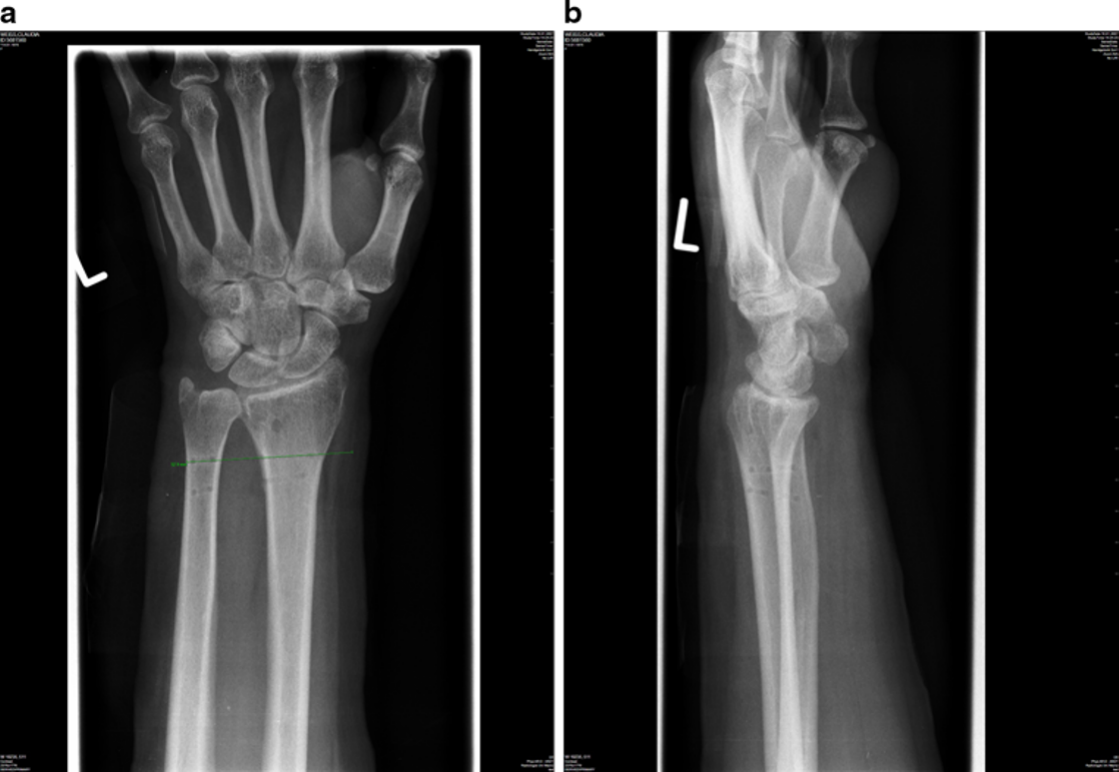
AP and lateral wrist radiographs showing proximal radial migration
